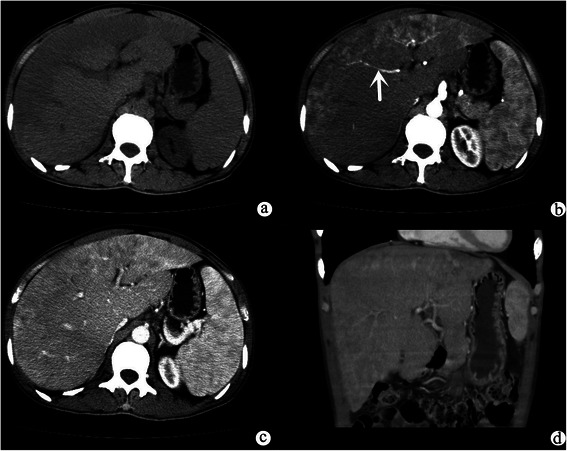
Axial unenhanced CT scan (a) showed lesions were homogeneous, lamellar, hypodense and subcapsular. Axial arterial phase (b) showed the lesions were heterogeneous enhancement, and a blood vessel tapered toward the lesions and was ended in the lesions (indicated by white arrow). Axial portal vein phase (c) showed the lesions were progressive enhancement. Coronal contrast-enhanced CT scan (d) showed the lesions were subcapsular
